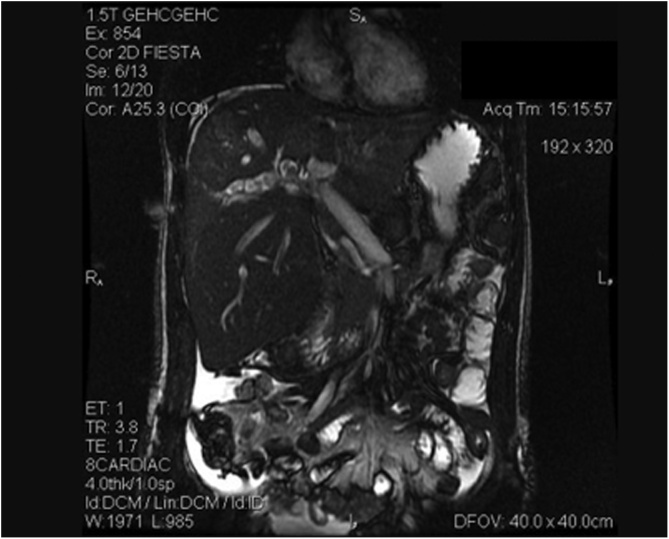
Preoperative MRI showing dilated intrahepatic bile ducts with stones inside.
